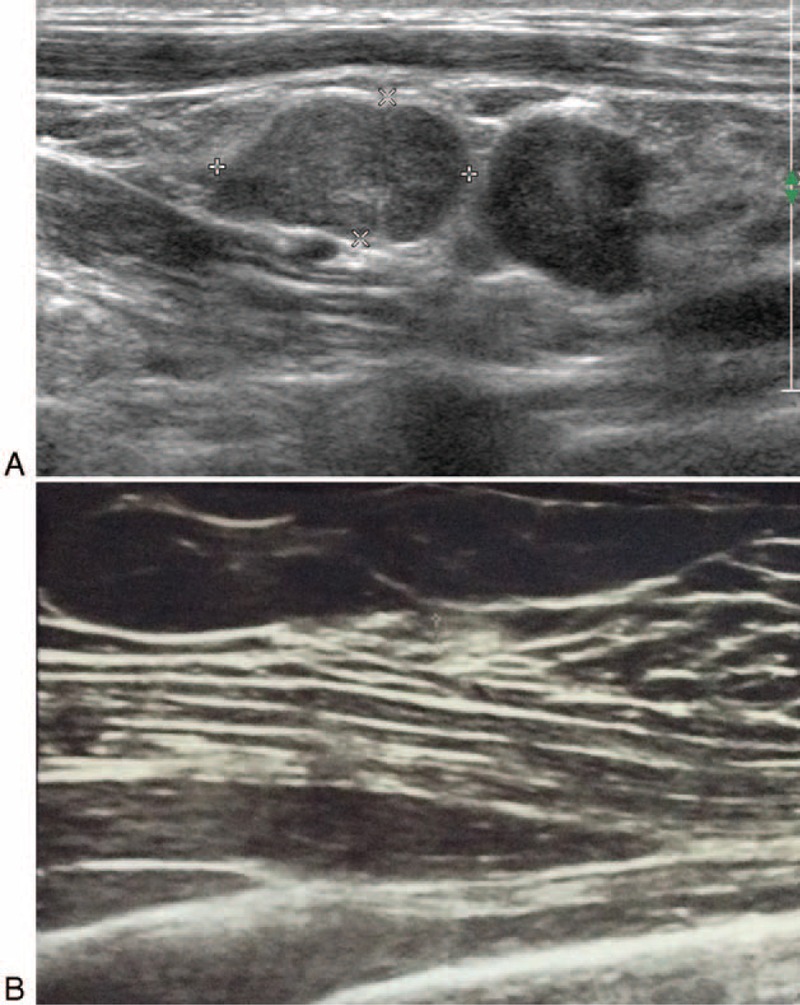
(A) US revealed enlarged abnormal lymph nodes approximately 2 cm in maximum size; they are round or oval shape with severe cortical thickening and displaced fatty hilum and increased parenchymal echogenicity in surrounding soft tissue. (B) Breast US of this patient revealed mammary gland manifests as proliferation of normal fatty tissue with maximum thickness of 1.8 mm. US = ultrasonography.
